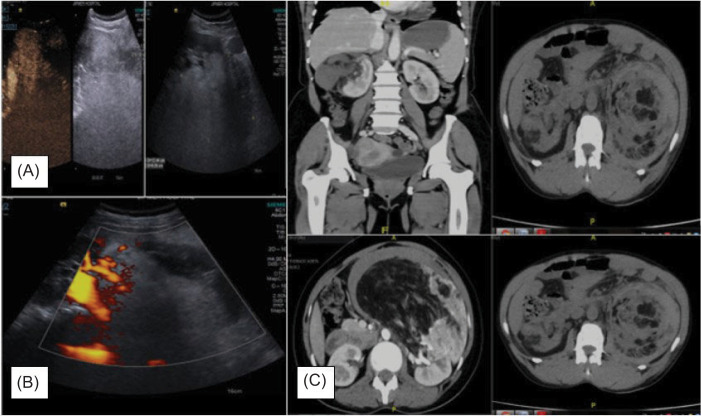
(A) Ultrasonographic images of renal angiomyolipoma; (B) Doppler images showing perirenal haemorrhage, that is, Wunderlich syndrome; (C) Contrast-enhanced computed tomography showing renal angiomyolipoma with Wunderlich Syndrome.
